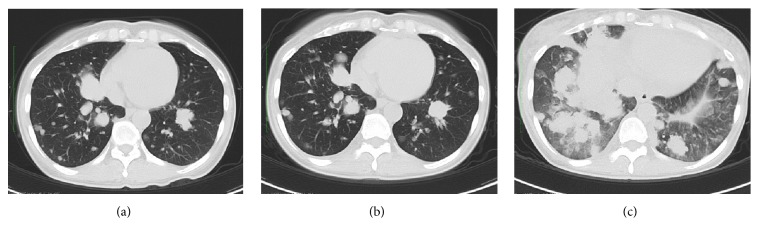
Transverse section of lung in computed tomography. (a) Start of pazopanib therapy. (b) End of pazopanib therapy. (c) After two weeks' cessation of pazopanib therapy.
